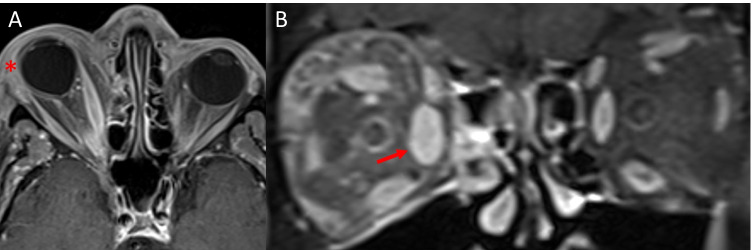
Orbital cellulitis. Axial fat-suppressed contrast-enhanced T1-weighted MRI (A) demonstrating preseptal soft tissue swelling (asterisks, *), enlargement and enhancement of the right medial rectus muscle and right axial proptosis. Coronal fat-suppressed contrast-enhanced T1-weighted MRI (B) demonstrates enlargement of the right medial rectus muscle (arrow) with associated intraconal fat stranding
